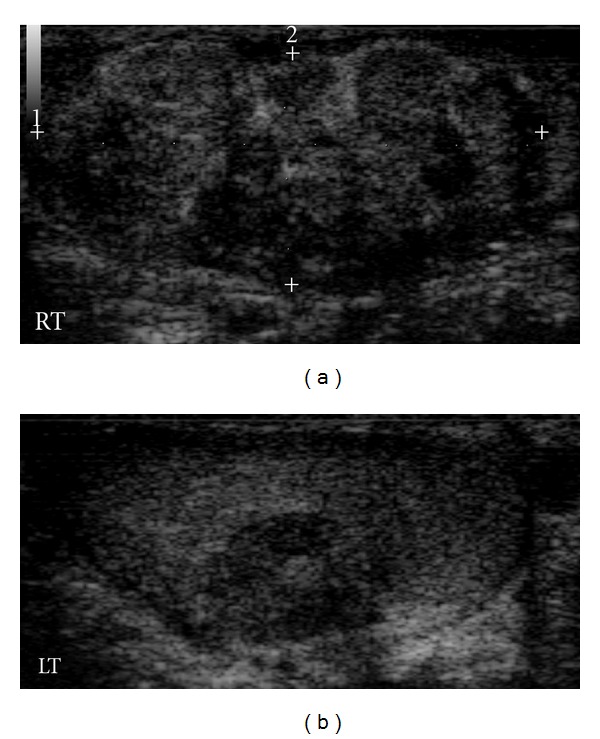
Longitudinal scrotal sonogram showing bilateral hypoechoic heterogeneous masses (RT: right testis, LT: left testis).
